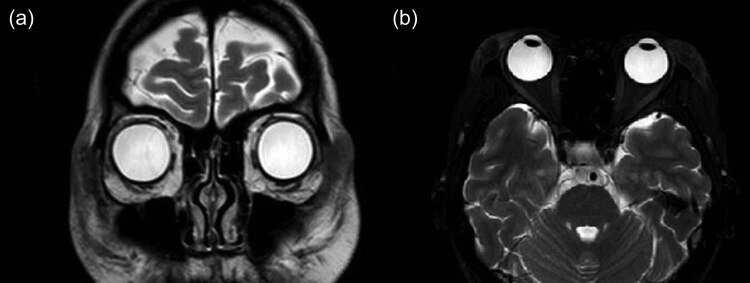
Contrast enhanced T2-weighted orbital MRI, showing thickened soft tissue around the orbit and enlargement of both lacrimal glands. (a) Coronal slice. (b) Horizontal slice.
